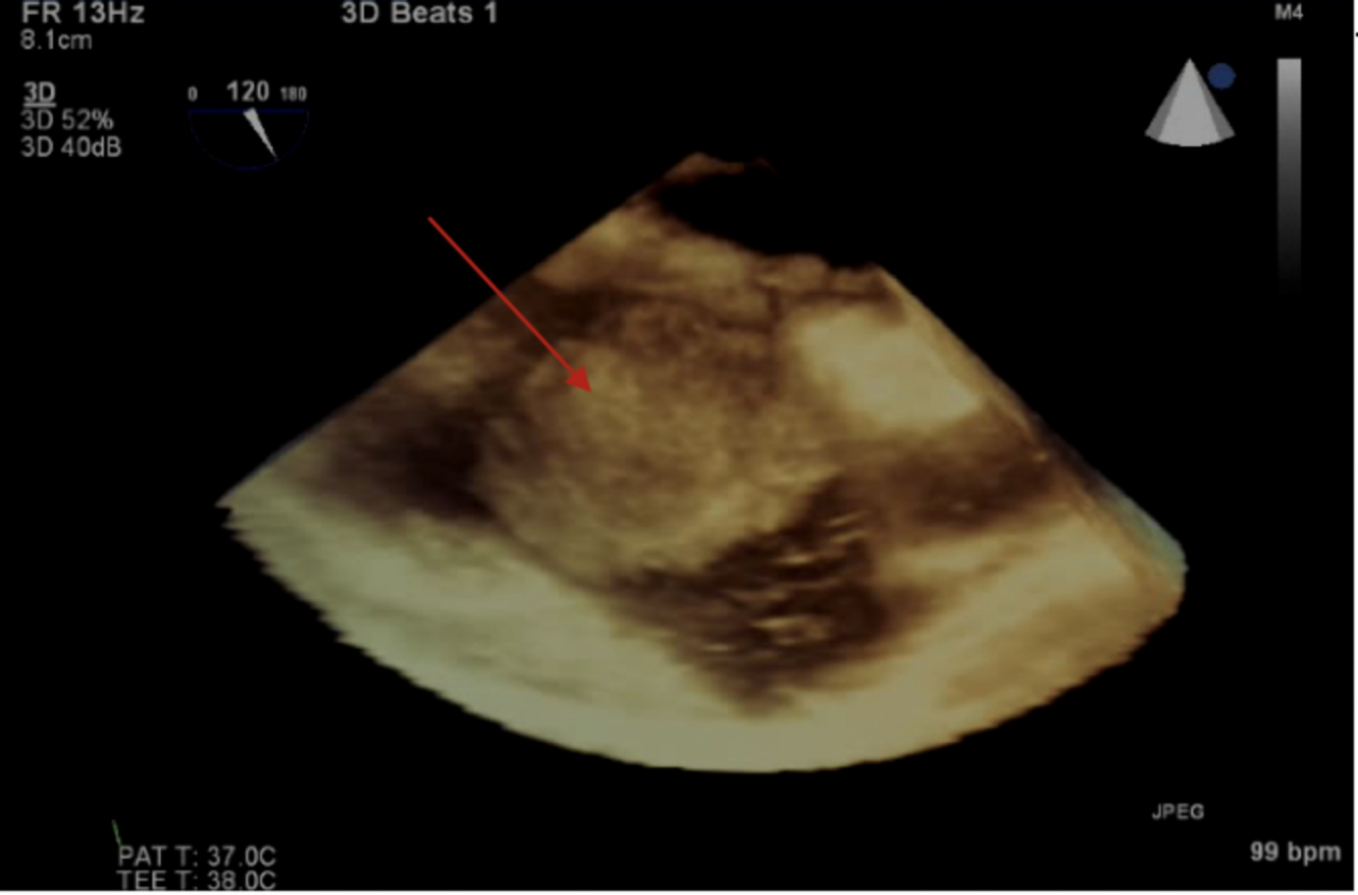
3D TEE showing right atrial mass (arrow). 3D TEE, three-dimensional transesophageal echocardiogram.
